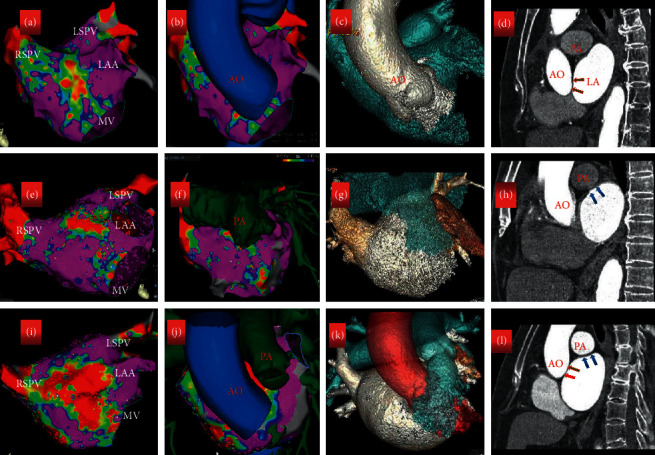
Fusion of the voltage map and the enhanced cardiac CT. Low-voltage area on the LAAW at the left atrium (LA)-aorta (Ao) (a–d) or LA-pulmonary artery (PA) (e–h) or both (i–l) contiguity. The three-dimensional (3D) electroanatomic voltage maps in anteroposterior views (a, e, i) and merged (b, f, j) with 3D enhanced cardiac computed tomography (CT) (c, g, k) corresponding images of enhanced cardiac CT (d, h, l). Ao:  aorta; LA: left atrium; PA: pulmonary artery; LAA: left atrial appendage; MV: mitral valve; LSPV: left superior pulmonary vein; RSPV: right superior pulmonary vein.
